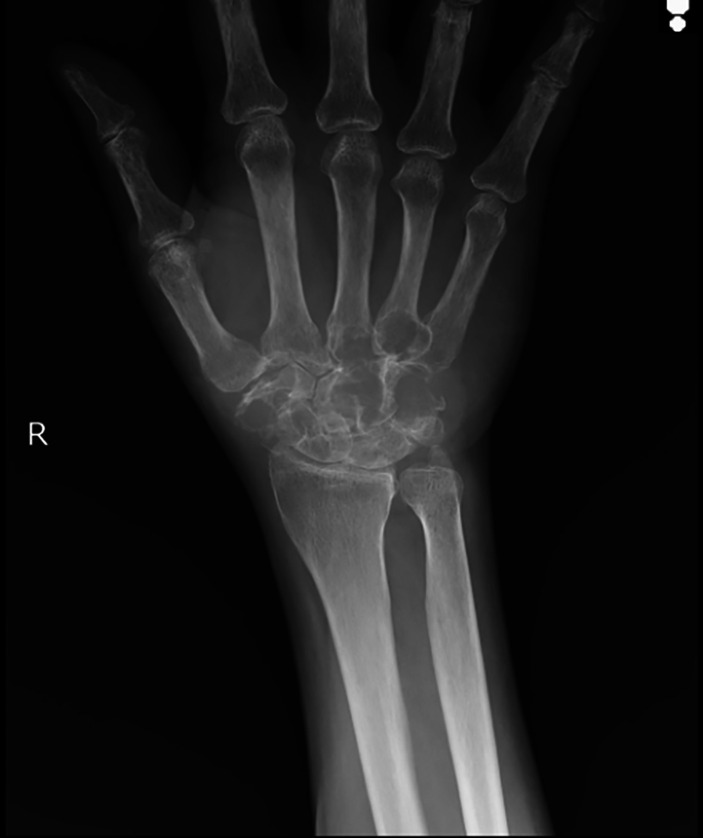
Anteroposterior X-ray right wrist demonstrated lytic lesions of carpal bones and base of 3
